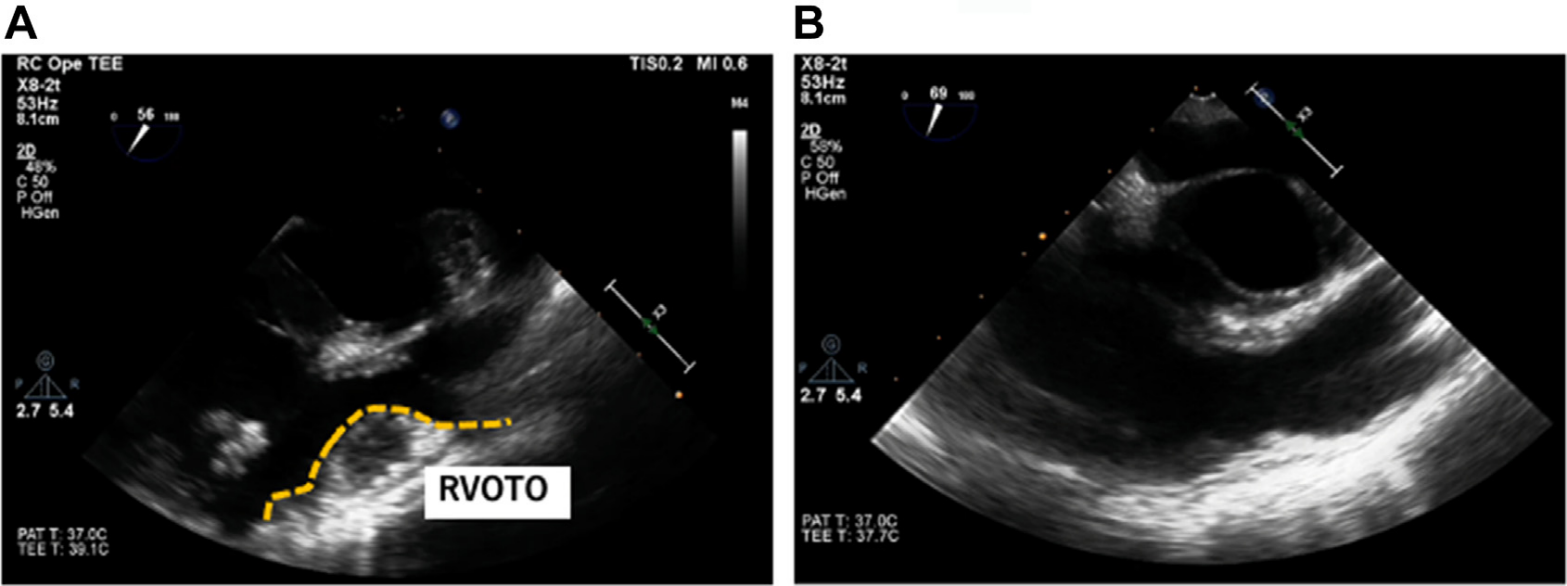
Transesophageal echocardiogram before operation (A), and after operation (B). (RVOTO, right ventricular outflow tract obstruction.)
